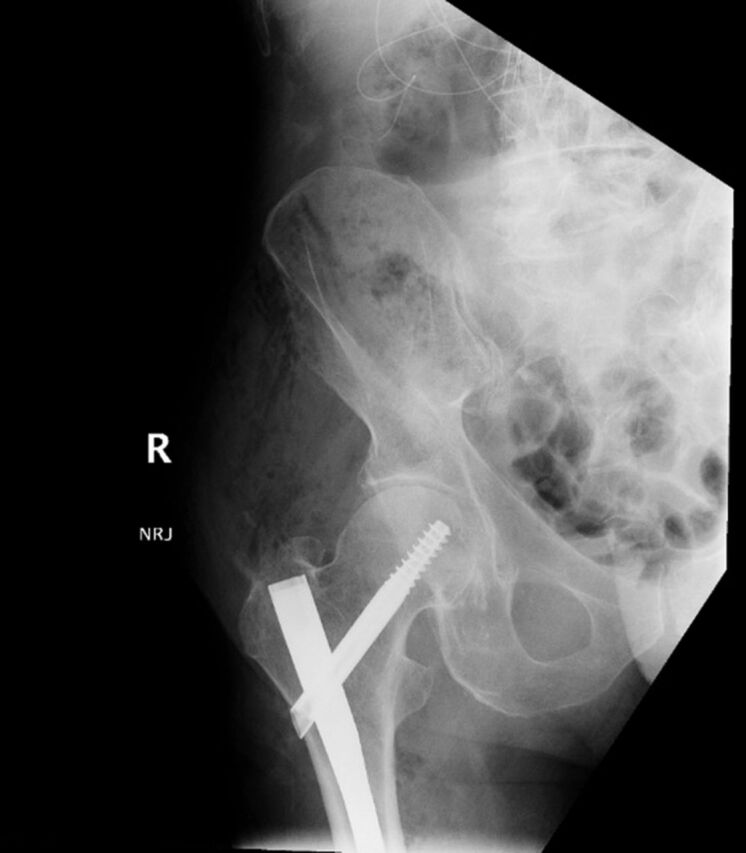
Postoperative anteroposterior x-ray of the right femur shows a cephalomedullary nail and a 100-mm hip screw.
